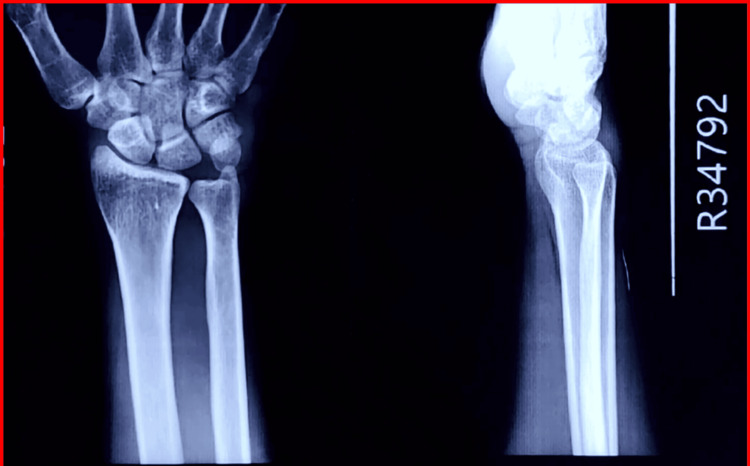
Anteroposterior radiograph of the wrist showing no bony abnormality
